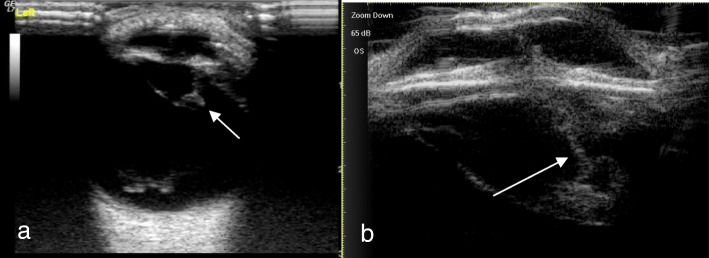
a The true positive image taken with 14-MHz + THI. The lens is hypoechoic and irregularly conical in shape, the PC is sharp, penetrating the vitreous body, and the rupture is moderately echoic (short arrow). b: The true positive LFUBM image. The ultrasonic performance is similar to that shown in a, with a medium echo penetrating channel in the crystal body (long arrow)
